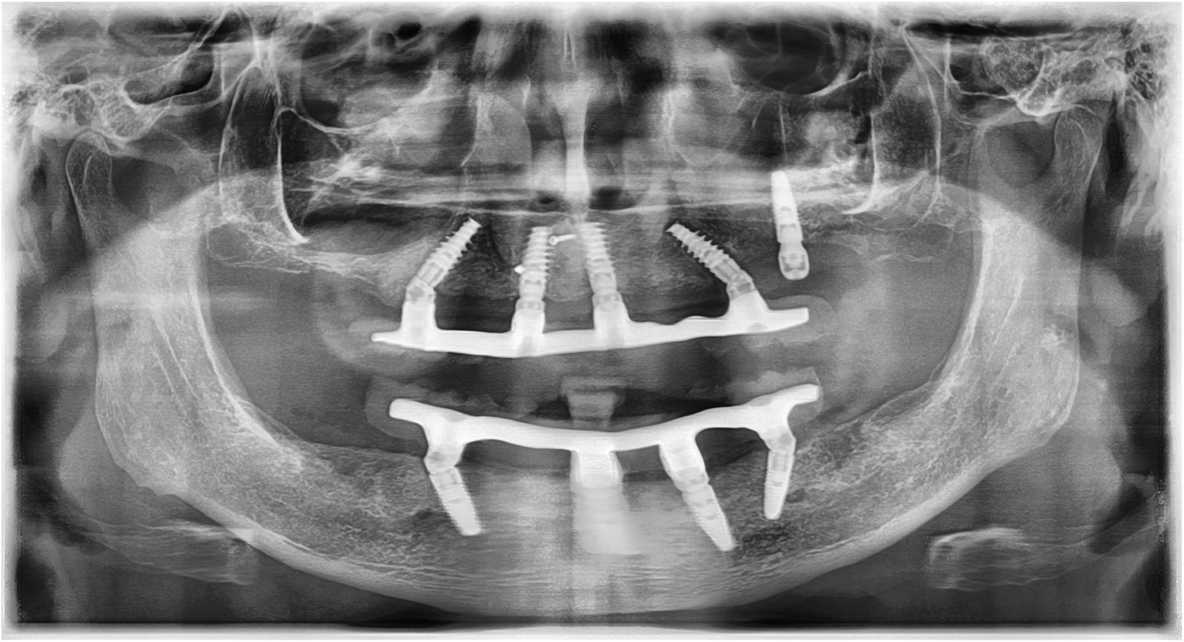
The procedure of what was done in case of an implant loss. The X-ray shows an All-on-4 restoration on four implants. The angled implant in the 2nd quadrant was loosened. For this reason, another implant with an external sinus elevation was placed in this area. During the entire healing period of this implant, the All-on-4 restoration was fixed on the remaining four implants, so that there was no load on the newly inserted implant
